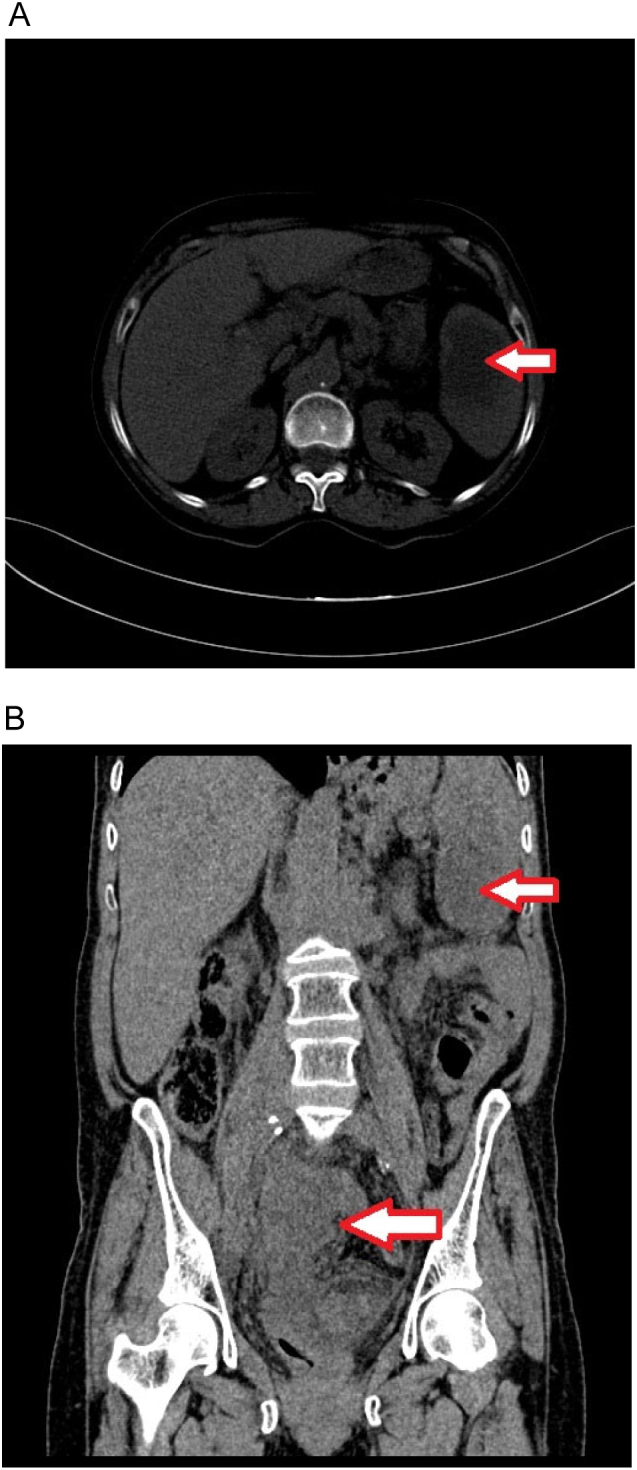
A. CT scan, axial view. The arrow shows a hypo-dense splenic mass. B. CT scan, coronal view. Arrows show a large uterine mass and a hypo-dense splenic mass.
